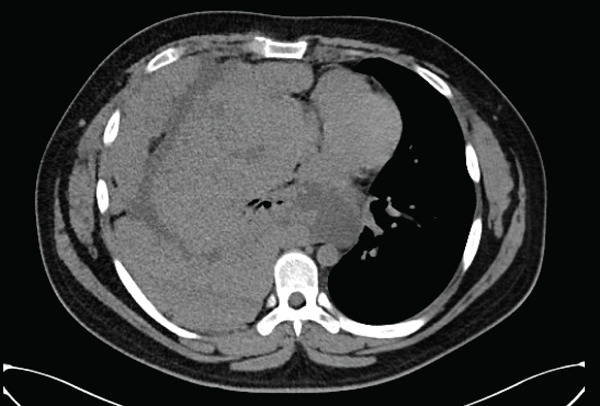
CT scan at initial diagnosis demonstrating extensive mass of the mediastinum and right hemithorax.
